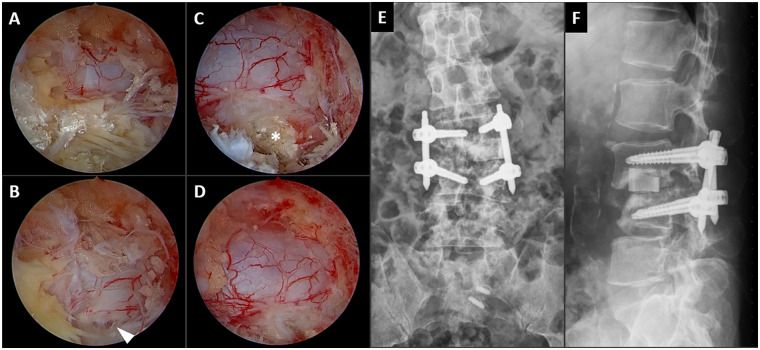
Intraoperative endoscopic images and postoperative plain radiography findings. Endoscopically, tophi material was observed mixed with the ligamentum flavum (A), and the complex was tightly adherent (arrowhead) to the dura mater (B). The herniated disc (asterisk) resulted in impingement of the right existing L3 nerve root (C). After completing the decompression, the right existing L3 nerve root and right transverse L4 nerve root got decompressed (D). The postoperative plain radiography showed that the kyphotic deformity of the L3−4 lumbar segment was corrected (E,F), and the image three months later revealed that the L3−4 lumbar segment was well fused.
